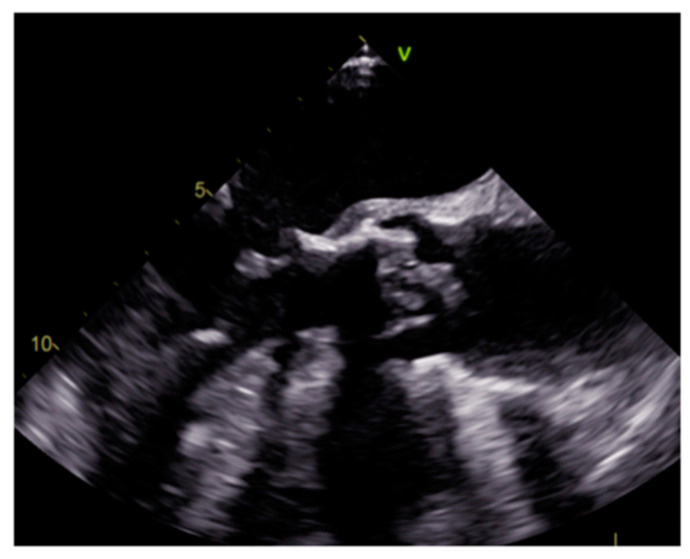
Prosthetic aortic valve infective endocarditis with vegetations at transesophageal echocardiography.
